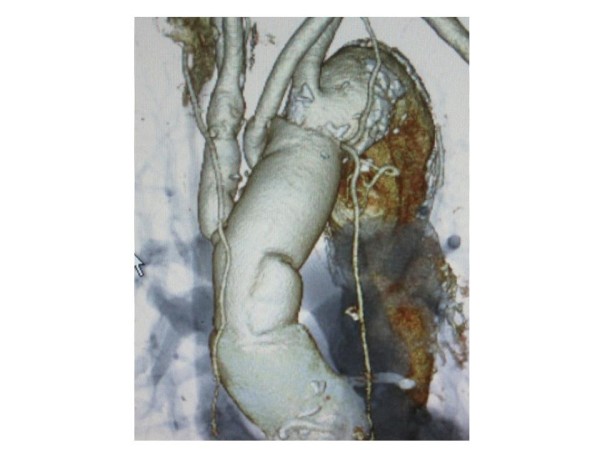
Postoperative 3-dimension computed tomography. There is no stenosis or dilatation of the branches.
